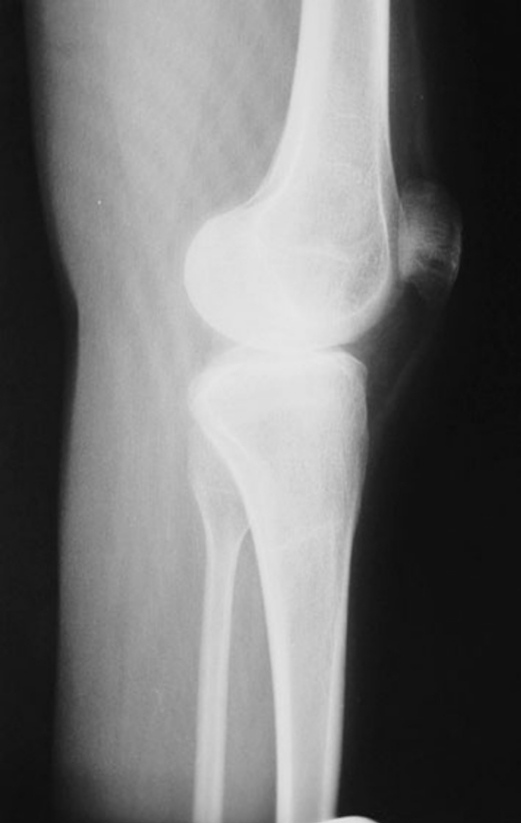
Preoperative plain X-ray showing minor flexion deformity of the knee (lateral view)
